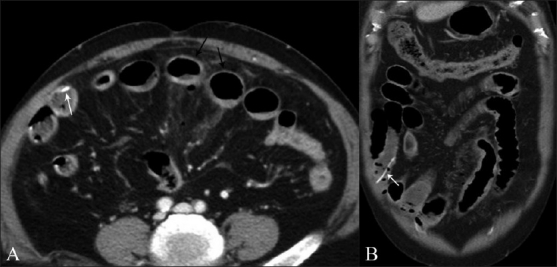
Small bowel perforation without obstruction. Axial (A) and coronal (B) contrast-enhanced CT scans show localized pneumoperitoneum and fat stranding in relation to focally thickened distal ileal loops (black arrows in A). The FB however, has since passed distally into the large bowel and can be easily overlooked on the axial images (white arrow in A). Note that the FB is fairly conspicuous on the coronal images (white arrow in B)
